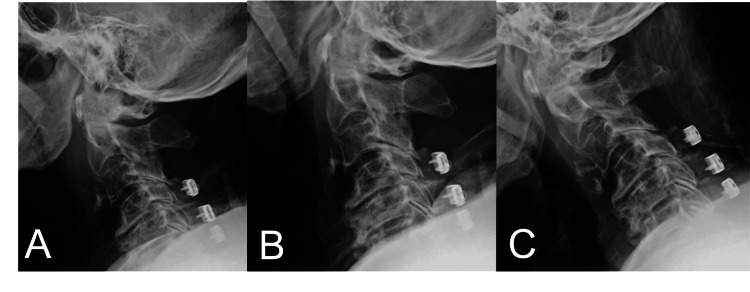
Dynamic radiographs taken one month after surgery show no instability of the cervical spine.
